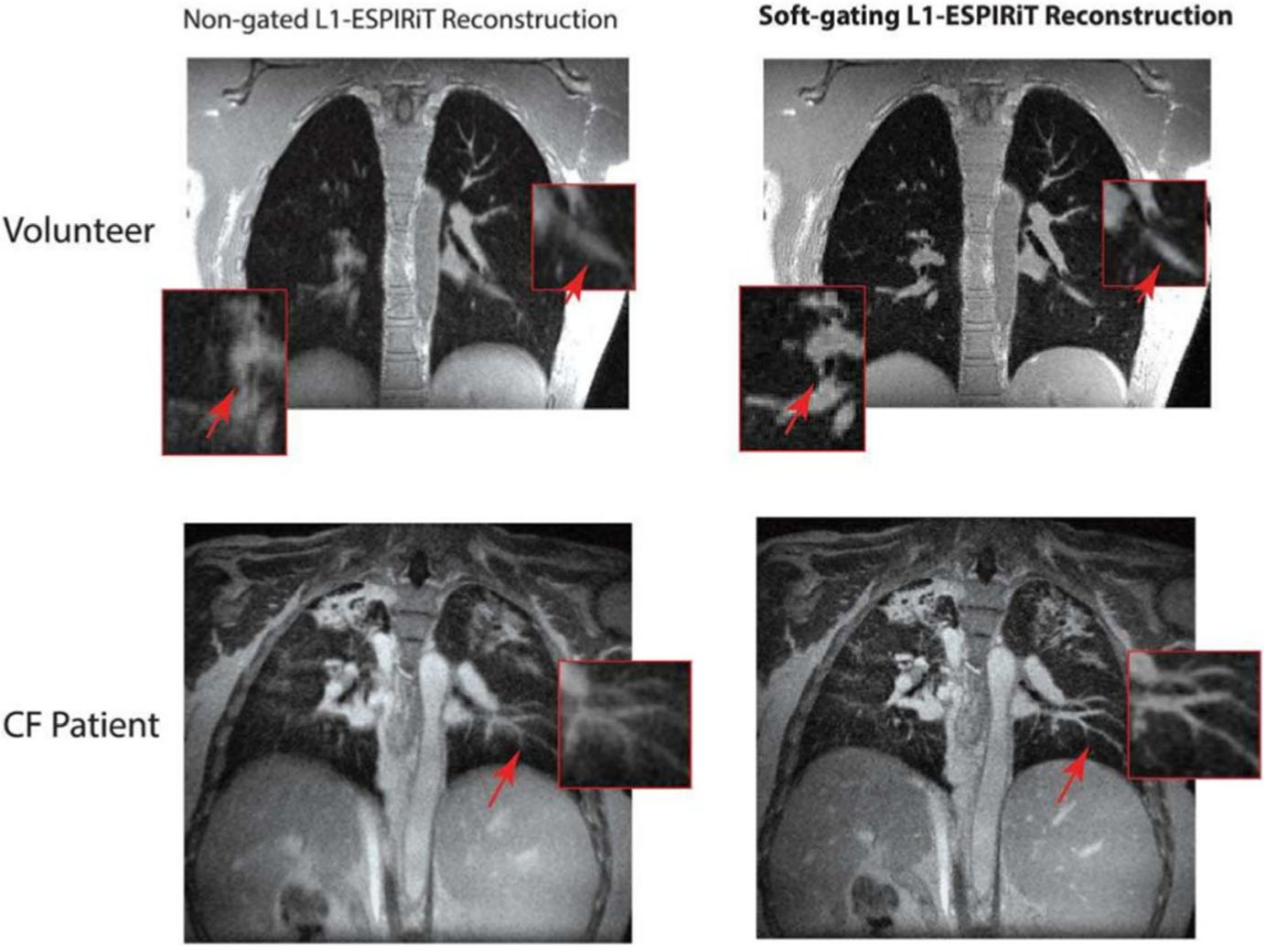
Demonstration of magnetic resonance imaging (MRI) respiratory blurring in both a healthy volunteer (top images) and a patient with cystic fibrosis (bottom images). Left versus right images show the improved performance after considering respiration within reconstruction. The MR images were determined using three‐dimensional ultrashort echo time sequences. Adapted from Jiang et al.^43^ [Color figure can be viewed at http://wileyonlinelibrary.com]
